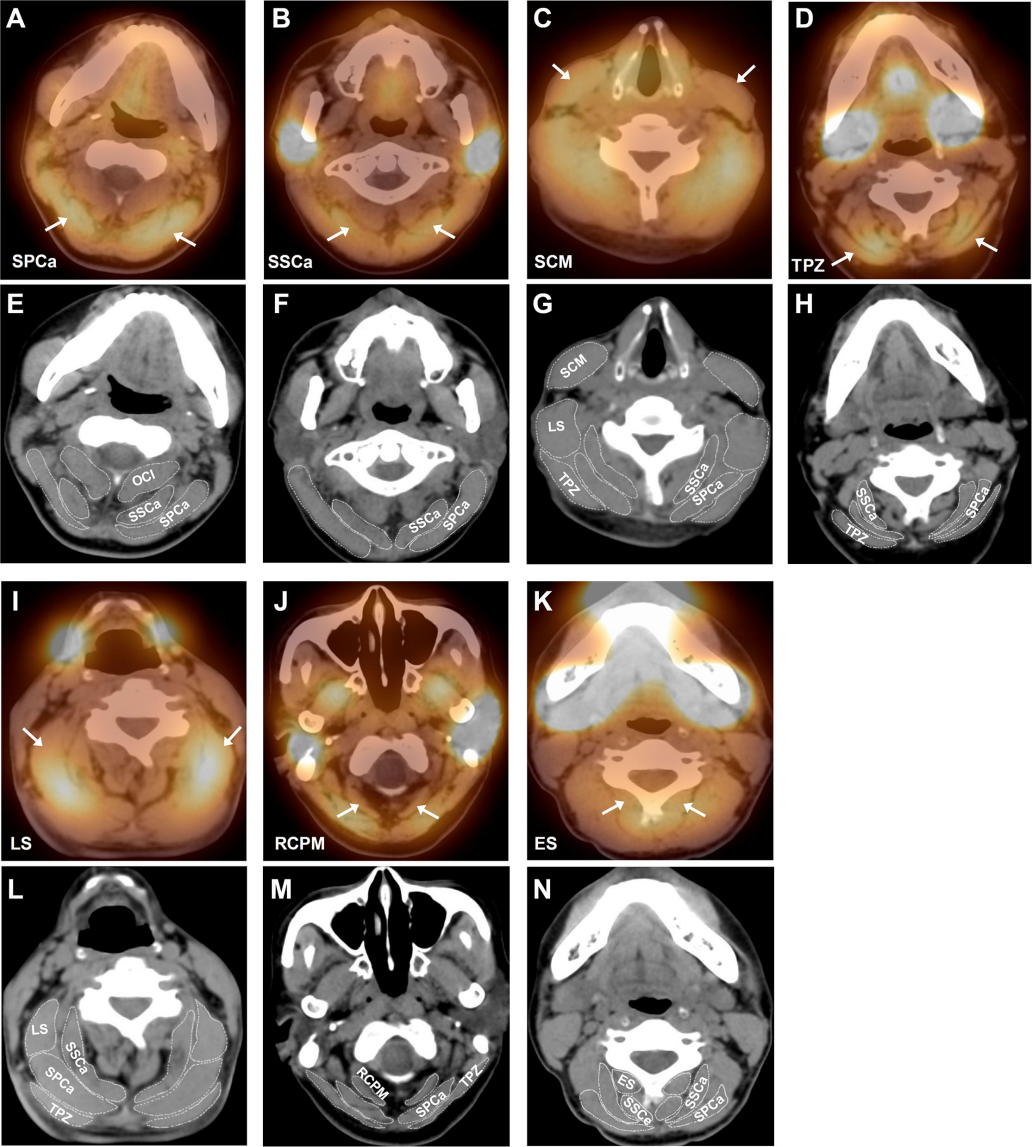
Representative SPECT/CT images of different activated cervical muscles in patients with RCD. (A) Bilateral activation of splenius capitis (SPCa) indicated by the white arrows; (B) bilateral activation of semispinalis capitis (SSCa) indicated by the white arrows; (C) bilateral activation of sternocleidomastoid muscle (SCM) indicated by the white arrows; (D) bilateral activation of trapezius (TPZ) indicated by the white arrows; (I) bilateral activation of levator scapulae (LS) indicated by the white arrows; (J) bilateral activation of rectus capitis posterior major (RCPM) indicated by the white arrows; and (K) bilateral activation of erector spinae (ES) indicated by the white arrows. To demonstrate the anatomy of cervical muscles more clearly, we put the CT images of the same level below each SPECT image and outlined the boundaries of related muscles. (E–H) are CT images of (A–D) respectively, and (L–N) are CT images of (I–K) respectively. OCI, obliquus capitis inferior; SSCe, semispinalis cervicis.
